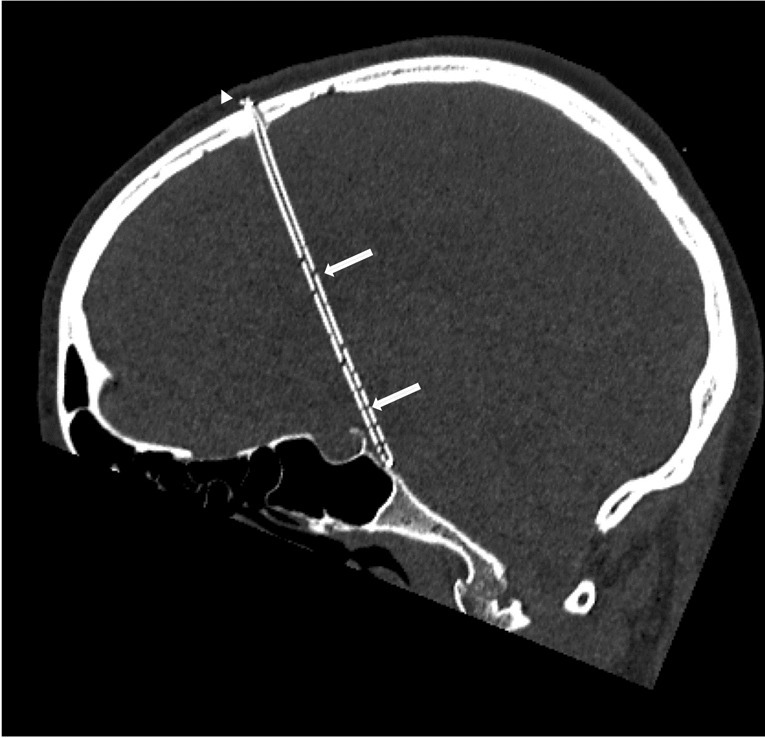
Postoperative CT scan of a patient treated with sTVIP. Bone window in a parasagittal oblique plane showing the entire length of the inner shunt. The catheter is fixed on the skull using a titanium clip (arrowhead) and fitted with appropriate drainage holes on the level of the lateral and third ventricle and the prepontine cistern (arrows)
